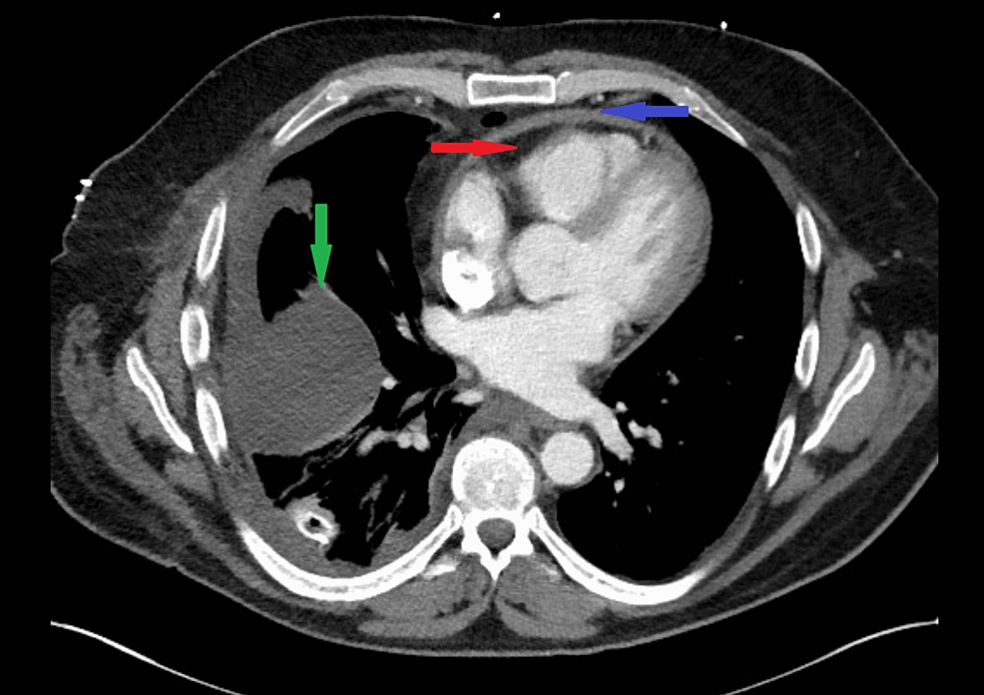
Chest computed tomography image showing pericardial thickening (blue arrow), small pericardial effusion (red arrow), and right-sided pleural effusion (green arrow).
